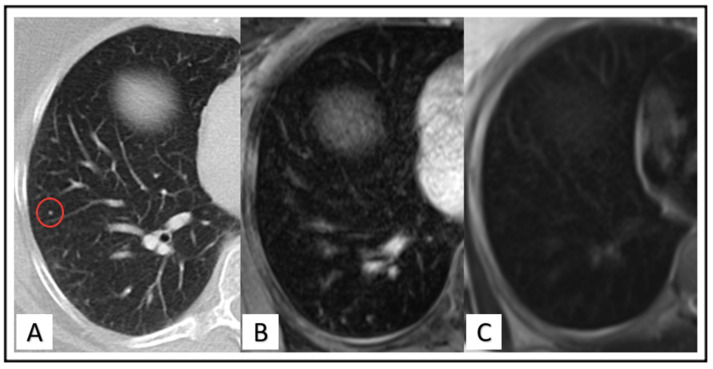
Images of a 46-year-old woman. Four-millimeter lung nodule located in right lower quadrant (red circle) identified on the CT image (A) but not recognizable in VIBE (B) nor HASTE (C) sequence.
